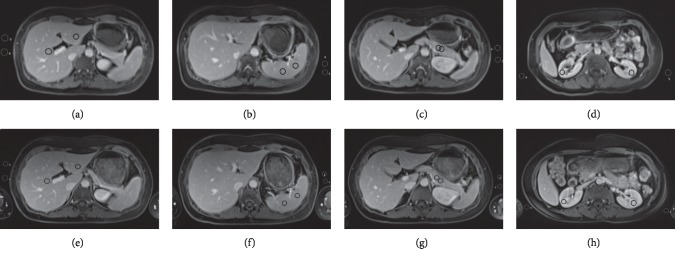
Abdominal visceral organ-enhanced images of a 56-year-old man. Equilibrium phase images of Gadobutrol-enhanced MRI: (a) liver (SNR 181.34, CNR 60.61); (b) spleen (SNR 202.06, CNR 109.39); (c) pancreas (SNR 161.11, CNR 54.65); (d) kidney (SNR 209.00, CNR 129.40). Equilibrium phase images of Gd-DTPA-enhanced MRI: (e) liver (SNR 145.56, CNR 44.48); (f) spleen (SNR 135.61, CNR 73.96); (g) pancreas (SNR 130.80, CNR 38.28); (h) kidney (SNR 187.62, CNR 101.84). The circles indicate ROIs.
